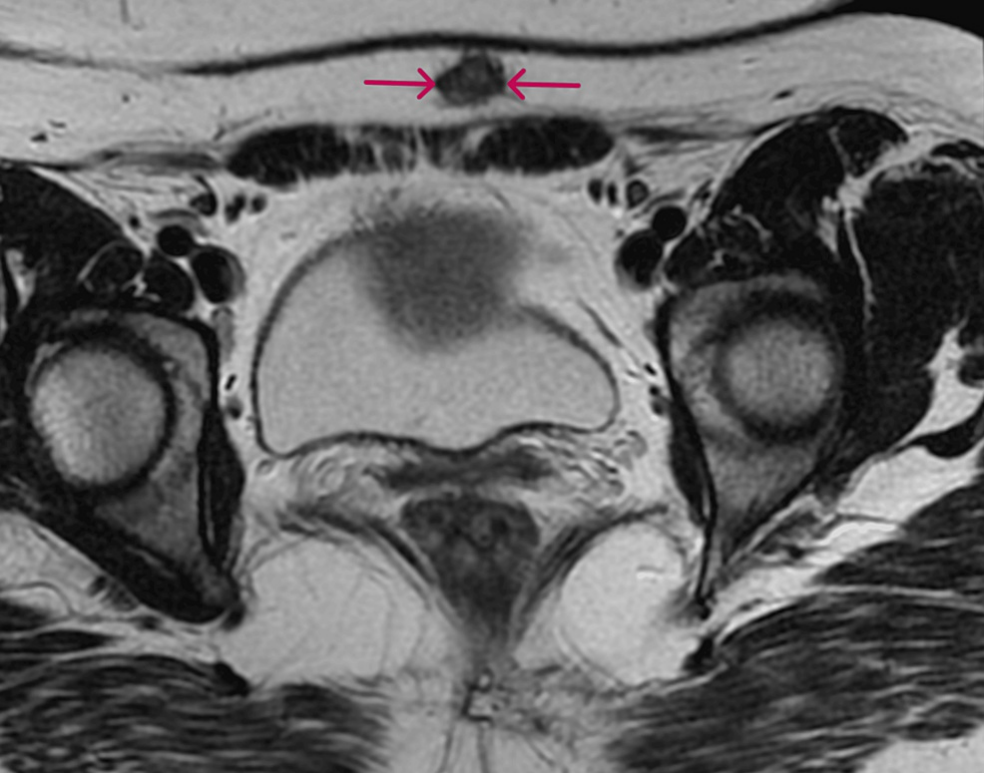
Magnetic resonance imaging of abdominal wall endometriosis Within the subcutaneous tissue, a solid spherical lesion (red arrows) is depicted, with the presence of vascularity
